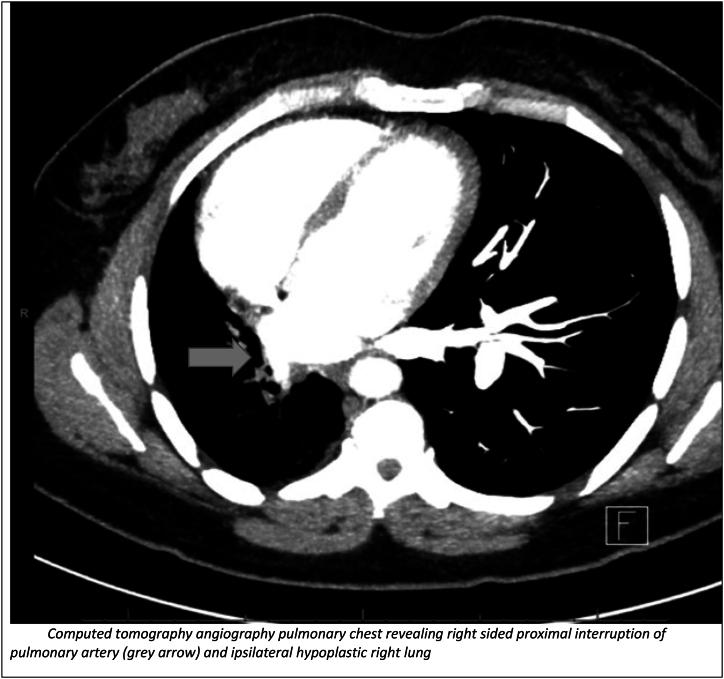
Computed tomography angiography pulmonary chest revealing right sided proximal interruption of pulmonary artery (grey arrow) and ipsilateral hypoplastic right lung.
